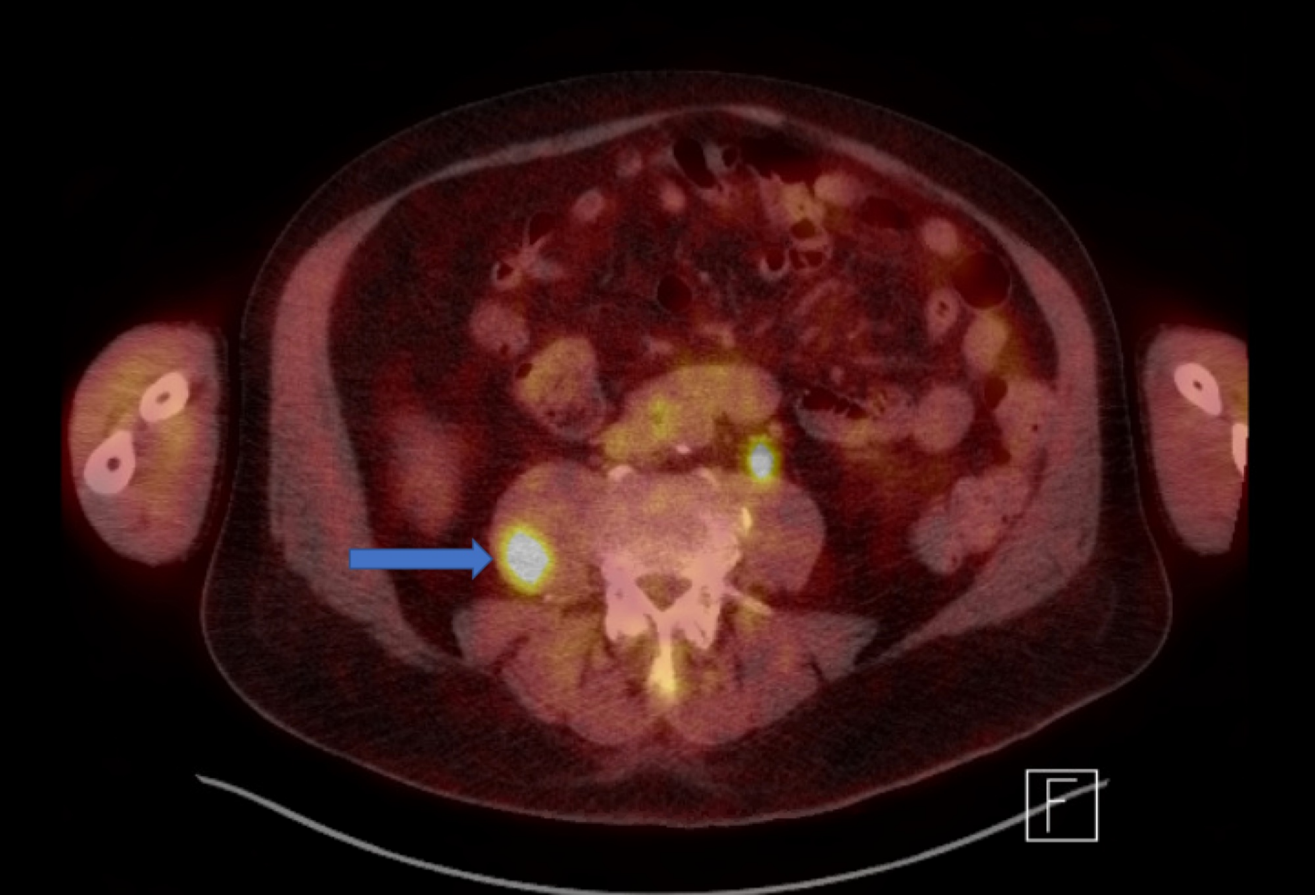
Positron emission tomography-computed tomography (PET-CT) demonstrating a 1.5-cm focus of FDG uptake in the right psoas muscle with an SUV max of 9.1 compatible with metastatic disease (arrow). FDG, F-fluorodeoxyglucose; SUV, standard uptake value.
